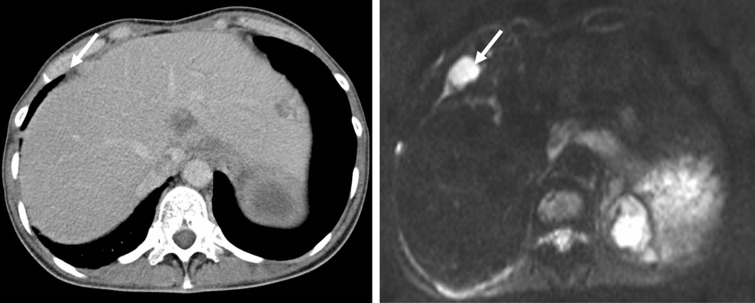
Peritoneal carcinomatosis from colorectal cancer in CT scan (left) and diffusion-weighted imaging (DWI), right. DWI shows lesions that are not visible in conventional CT scan (arrow).
